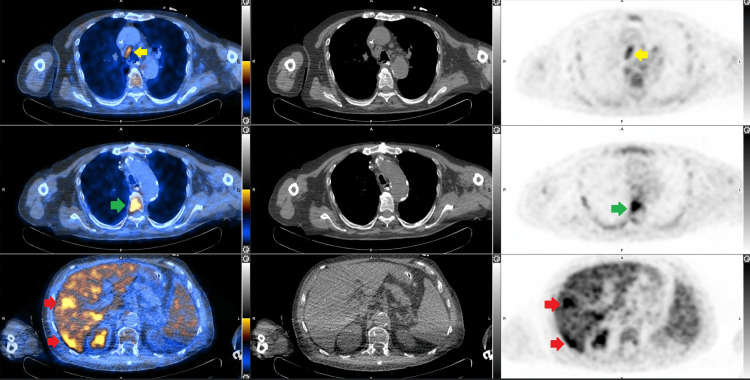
Fused F-18-FDG PET/CT, CT scan, and F-18-FDG PET of the abdomen and thorax The axial sections show hypermetabolic mediastinal lymph nodes (yellow arrow), hypermetabolic activity at the T5 vertebral body (green arrow), and hypermetabolic activity in the right liver (red arrows). F-18-FDG: Fluorine-18 fluorodeoxyglucose
